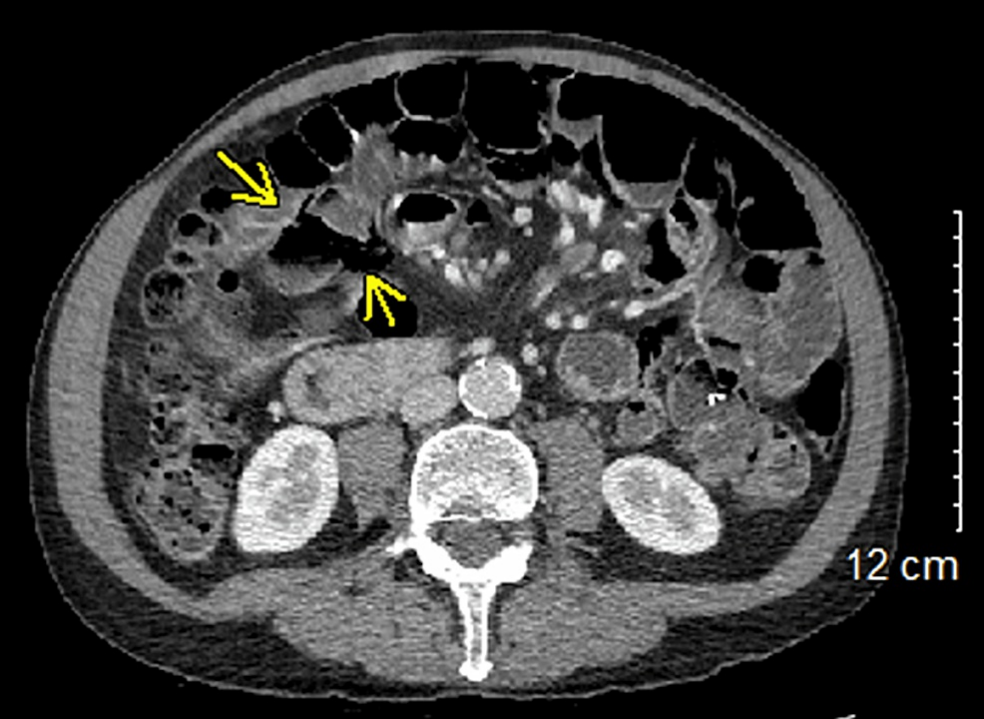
Axial contrast-enhanced abdominal CT showing an abnormal loop of small bowel in RUQ (yellow arrows) with diffuse pneumatosis CT: computed tomography; RUQ: right upper quadrant
